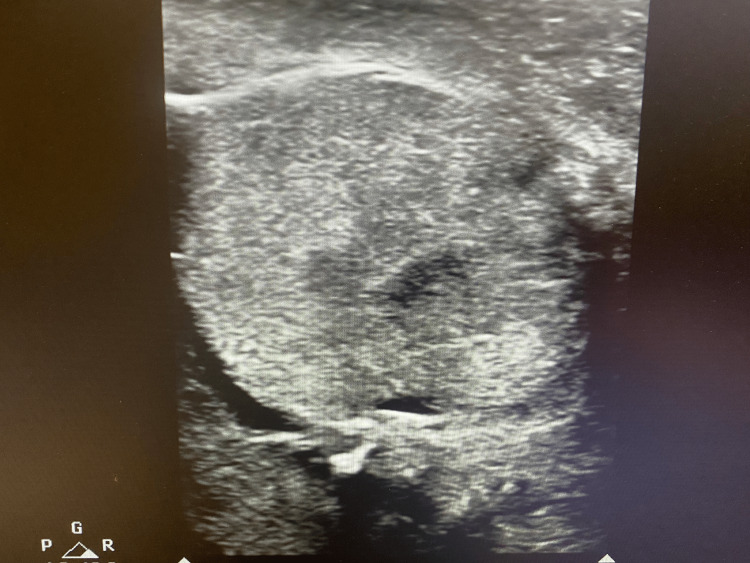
Ultrasound of the left testis revealing a decrease in the size The figure is presented with the patient’s informed consent.
